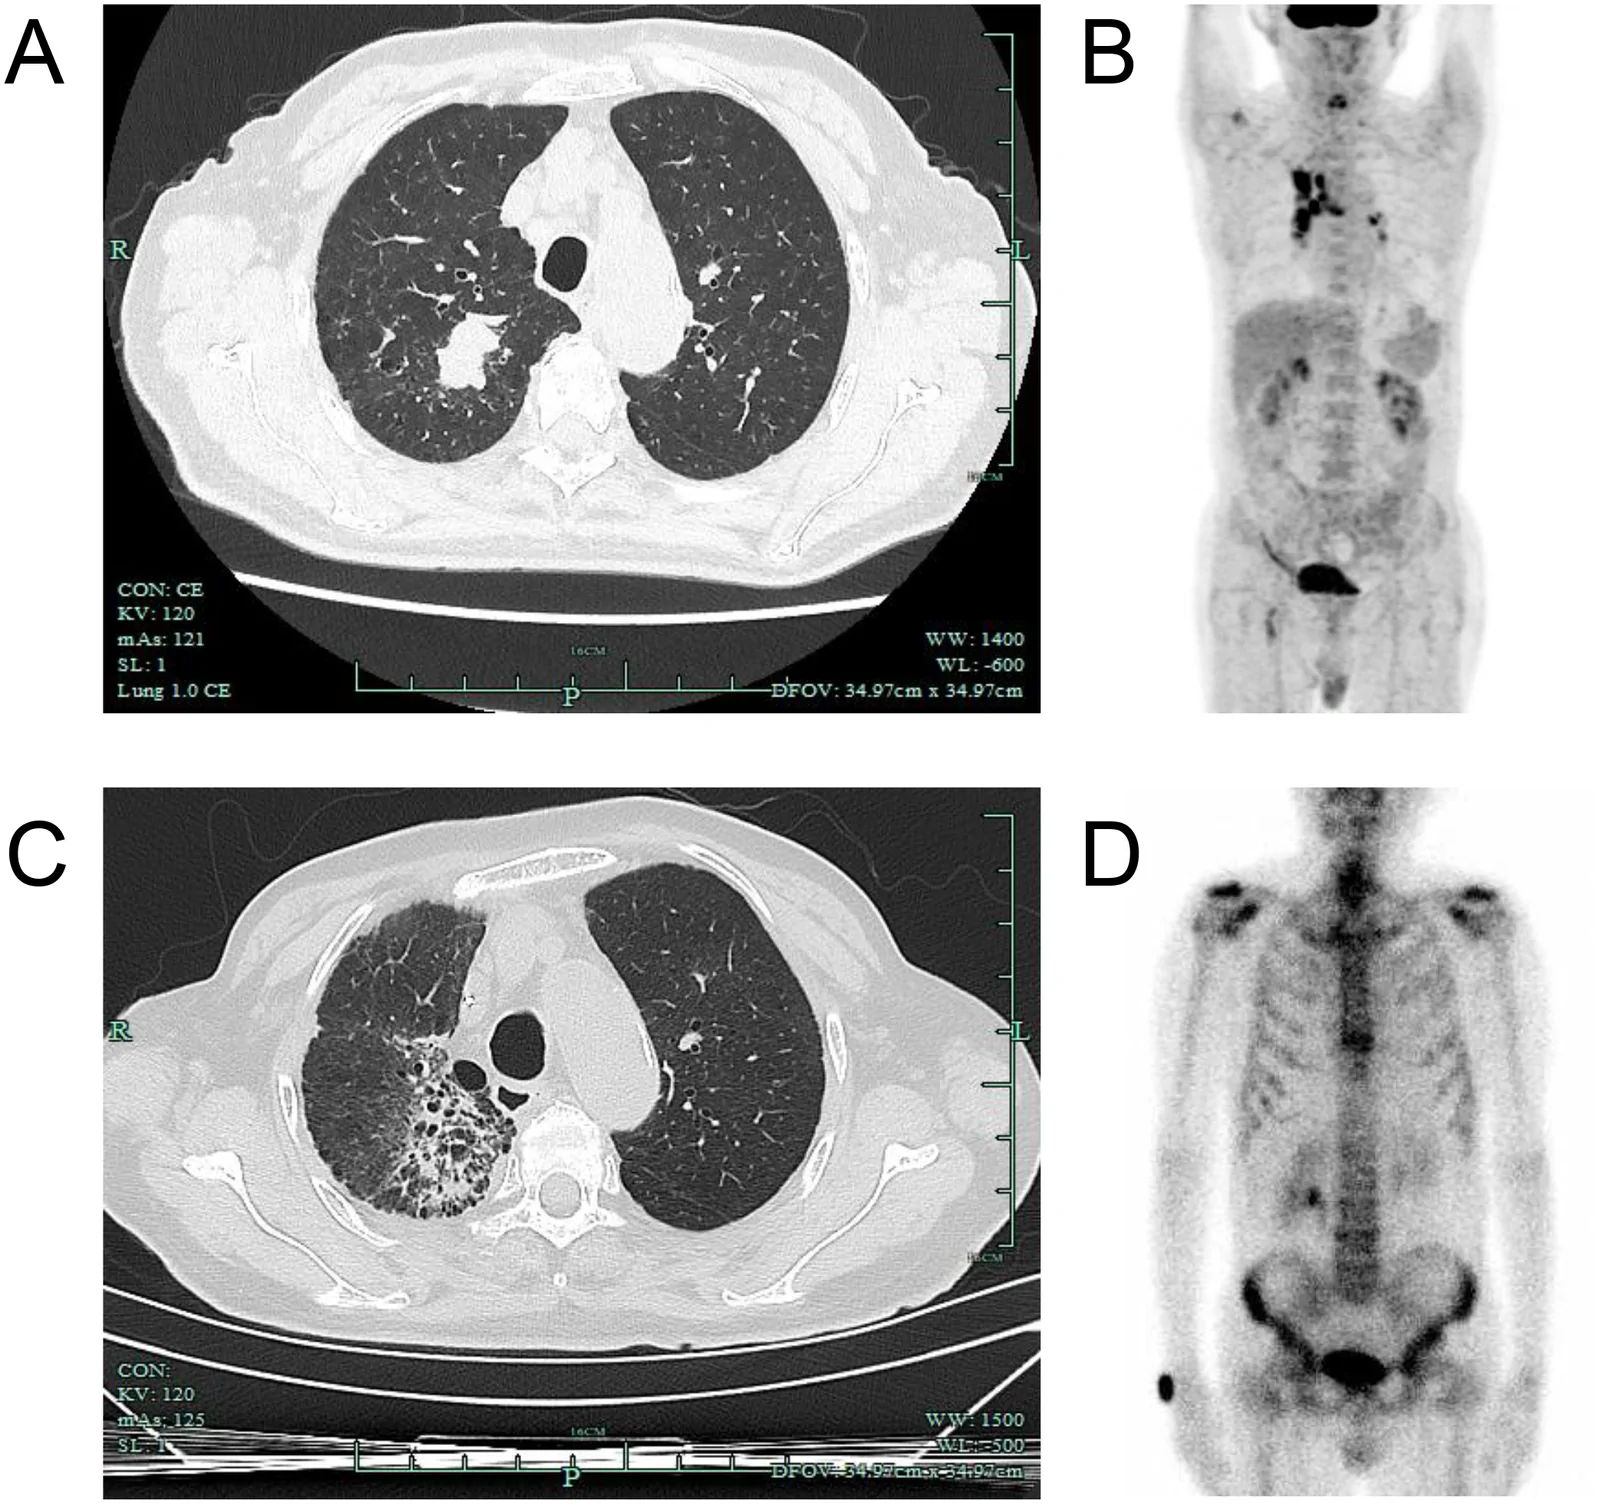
Radiological findings at initial diagnosis and terminal admission. (A) Initial contrast-enhanced chest CT showed a right upper-lobe lesion. (B) Initial whole-body PET/CT showed hypermetabolic uptake in the right upper-lobe lesion and regional lymph nodes, without definite distant or skeletal metastasis. (C) Chest CT during the terminal admission showed post-treatment changes without obvious enlargement of the primary lesion. (D) Bone scintigraphy during the terminal admission showed no definite bone metastasis.
